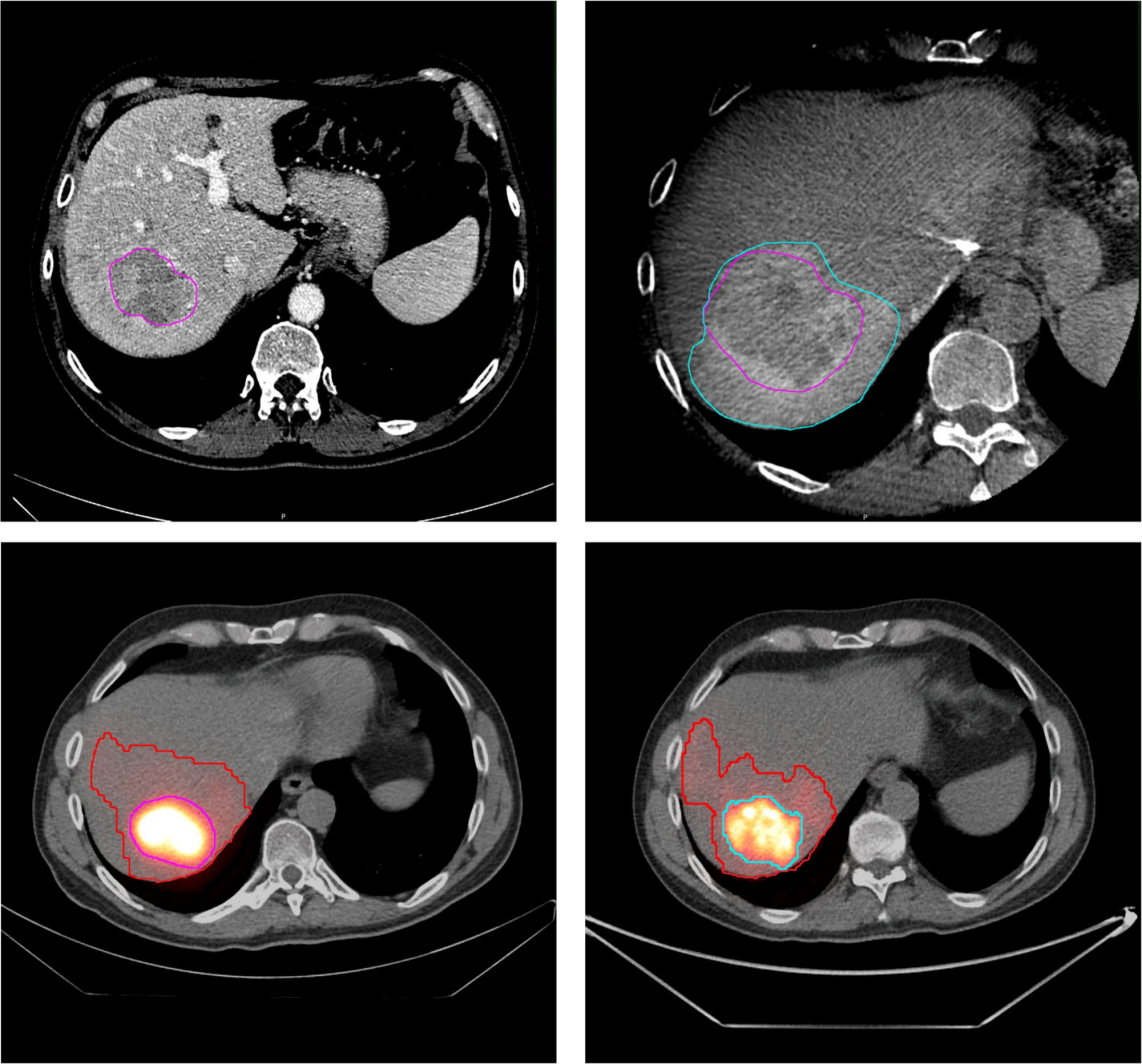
TV and PV segmentation in different imaging modalities a) CECT portal phase, b) CBCT late arterial phase c) 99mTc-MAA-SPECT, d) 90Y PET/CT
